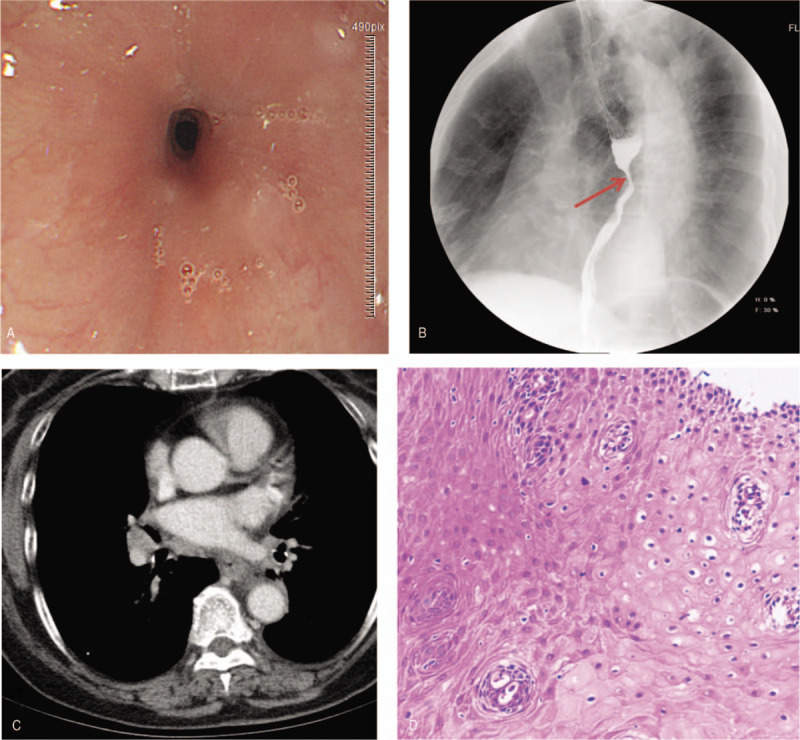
(A) Gastroscopy demonstrated concentric narrowing of the esophageal lumen at 30 cm from the central inciso. The mucosa overlying the stricture was smooth.(B) Barium esophagography showed a narrowing of approximately 1.5 cm in length in the middle and lower esophagus without visible disruption of the mucosa.(C) CT revealed that the wall of the middle and lower esophagus was obviously thickened.(D) The biopsy results suggested squamous epithelial hyperplasia.
